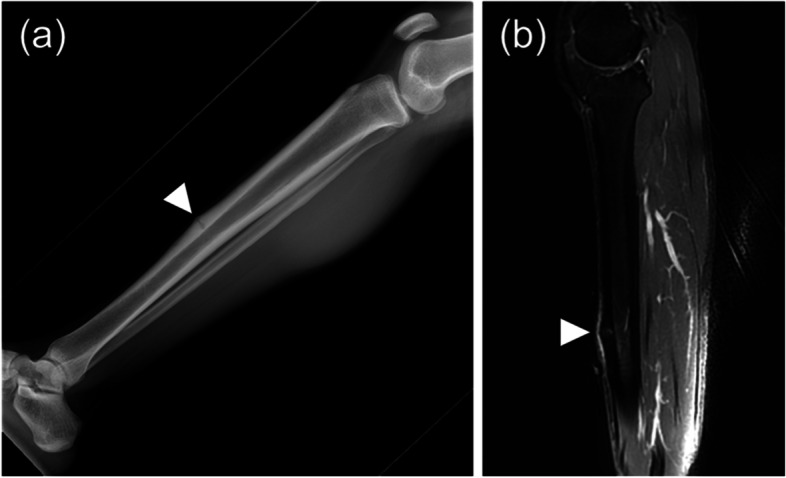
Medial tibial stress syndrome evaluated as Grade 4b in the Fredericson classification. a The lateral view of the lower leg X-ray demonstrated cortical thickening and fracture line of the tibial diaphysis (arrow). b MRI showed abnormal signal intensity in the tibial cortex and bone marrow oedema on STIR (arrow)
